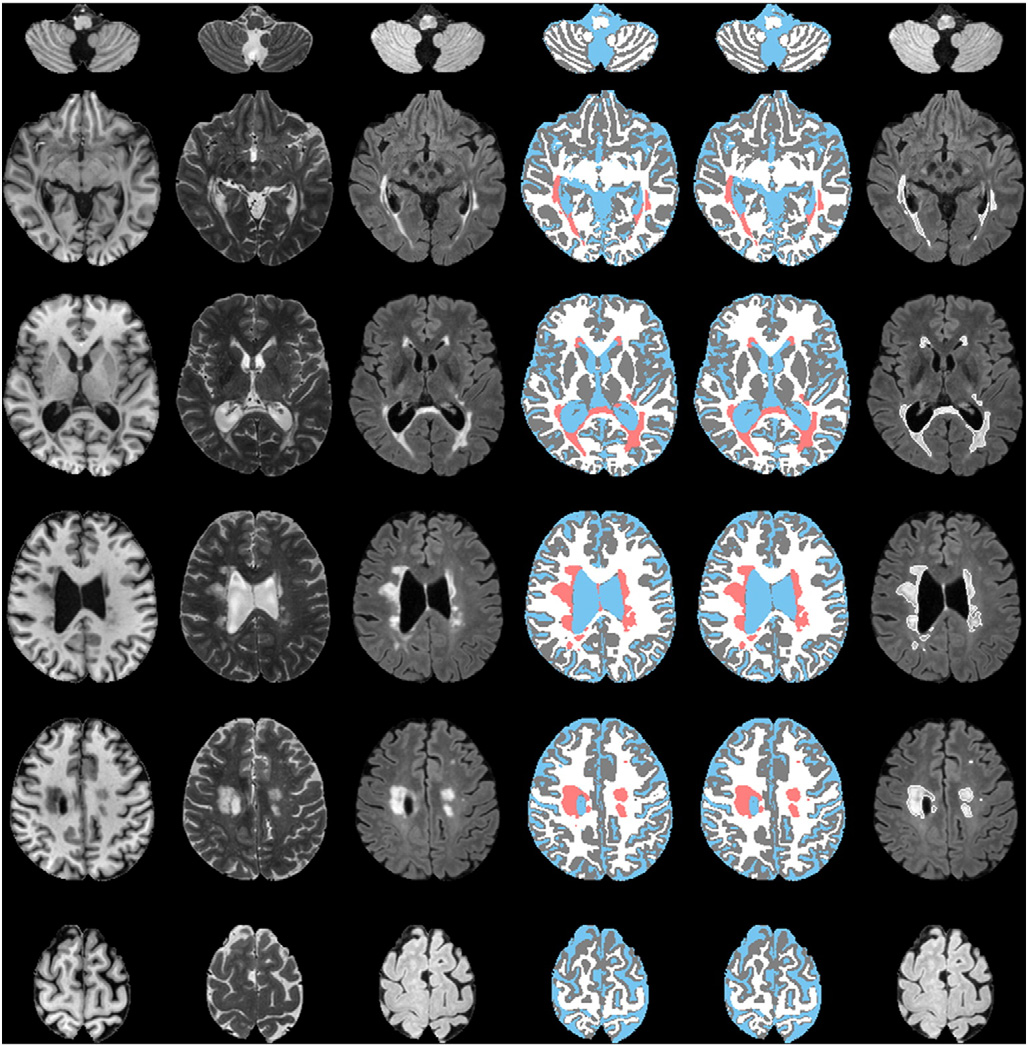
Automated segmentation at various cross-sections from one subject. First column: T1; second column: T2; third column: FLAIR; fourth column: segmented; fifth column: images with corrected lesions; and sixth column: boundaries of corrected lesions superimposed on FLAIR images. GM, WM, CSF, and lesions are represented by gray, white, blue, and salmon colors.
